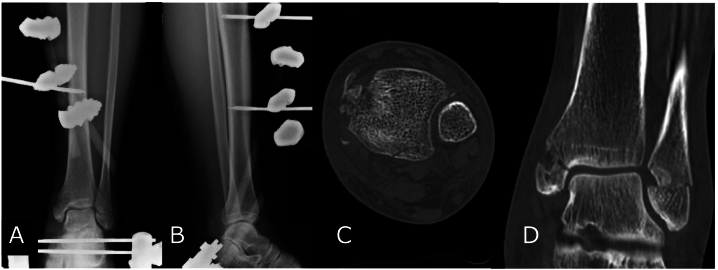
X-rays and CT after the reduction. A) Anterior posterior view of X-ray of the lower leg. B) Lateral view of X-ray of the lower leg. C) Axial view of CT showed posterior malleolar fracture. D) A compression fracture was identified proximal to the Weber type A distal fibular fracture.
